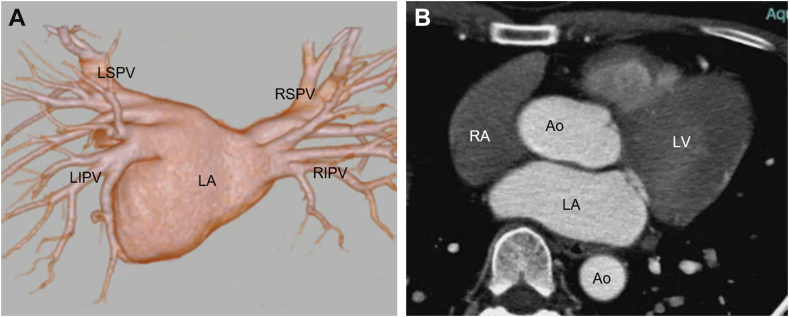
Cardiac CT of the Left Atrium and Pulmonary Veins (A) Posterior view showing normal anatomy of the left atrium and pulmonary veins. (B) The left atrium is not dilated. Ao = aorta; LA = left atrium; LIPV = left inferior pulmonary vein; LSPV = left superior pulmonary vein; LV = left ventricle; RA = right atrium; RIPV = right inferior pulmonary vein; RSPV = right superior pulmonary vein.
